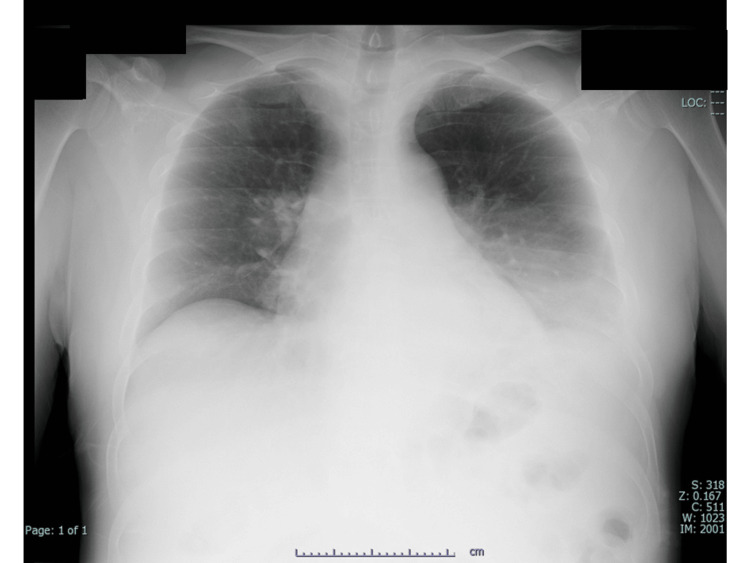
Chest X-ray showing bilateral lower lung consolidation.
